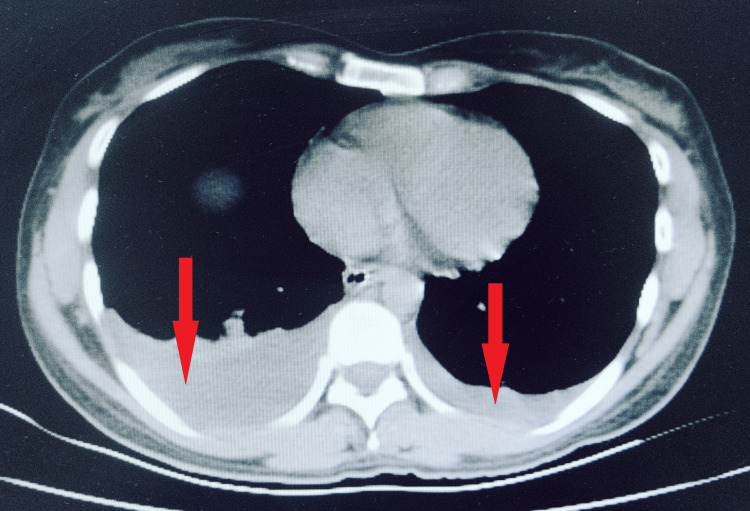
CT image showing bilateral pleural effusion marked by arrows
